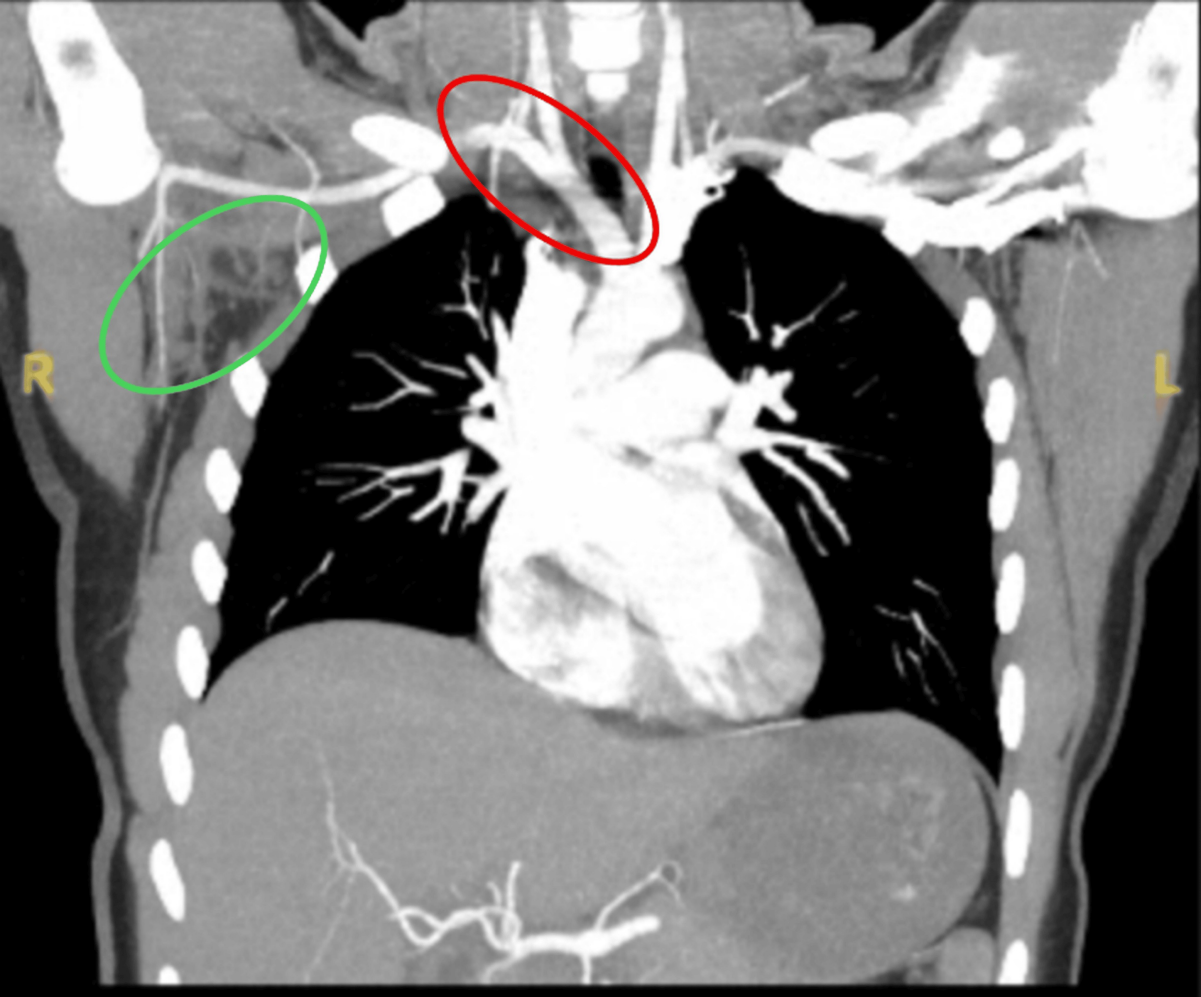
CT angiogram showing dilated, hyperdense subclavian and axillary veins indicated by the red oval with mild surrounding fat stranding indicated by the green oval, consistent with acute thrombus
